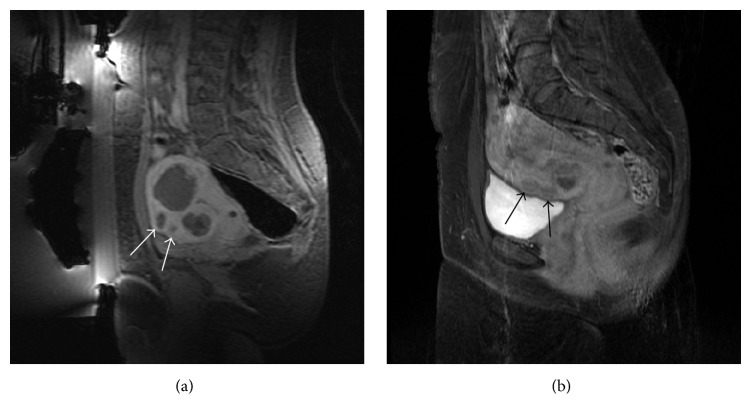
Case 1: (a) sagittal T1-w with fat saturation acquired after intravenous injection of paramagnetic contrast medium showing the two small fibroids (white arrows) located in the near field that were not directly treated but that became nonperfused too after the treatment; two bigger fibroids treated are visible too; (b) a follow-up MRI (T1-w with fat saturation acquired after intravenous injection of paramagnetic contrast medium) showing a normal vascularization of the two small fibroids.
